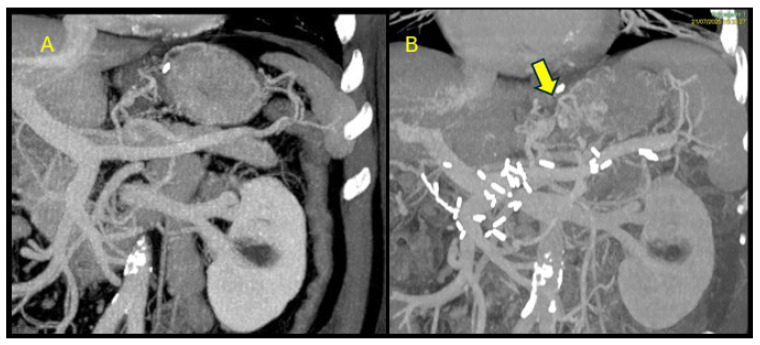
Coronal imaging. Comparison between pre-operative (A) and post-operative (B) CT scans. The arrow indicates the iatrogenic portosystemic shunt between the portal territory and the azygous vein.
